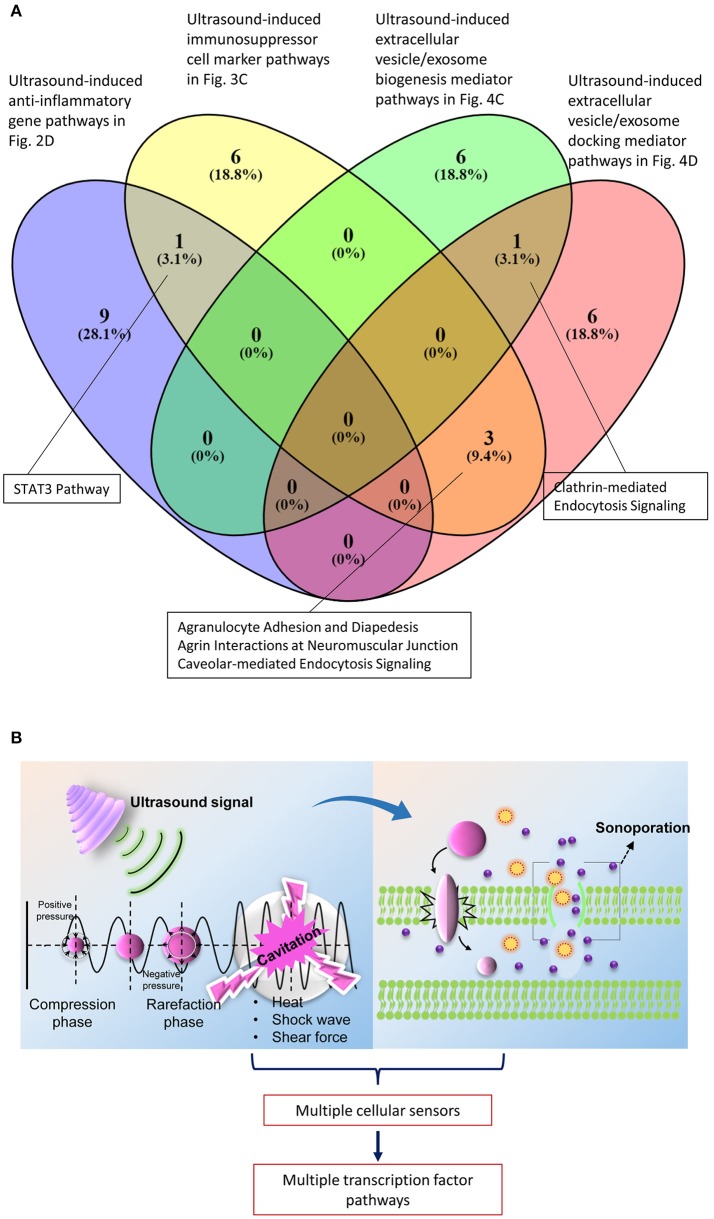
Most of the signaling pathways that are regulated by the mechanisms that are activated by LIUS are not shared. (A) Venn analysis revealed that STAT3 signaling pathway is shared between the anti-inflammatory genes and immunosuppressive regulator genes that are upregulated by LIUS therapy. Likewise, clathrin mediated endocytosis signaling is shared by genes that enhance exosome biogenesis and docking with ultrasound therapy and 3 signaling pathways are shared by immunosuppressor regulator genes and exosome docking genes. (B) The physical properties exerted by ultrasound therapy may activate multiple cellular sensors that activate distinct transcription factors to regulate various signaling pathways.
